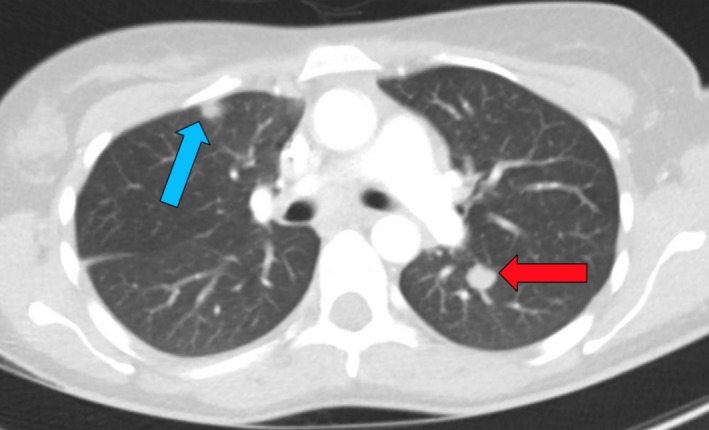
CT scan of chest identifying a pulmonary lesion (red) and an infarct (blue) due to a pulmonary embolus
